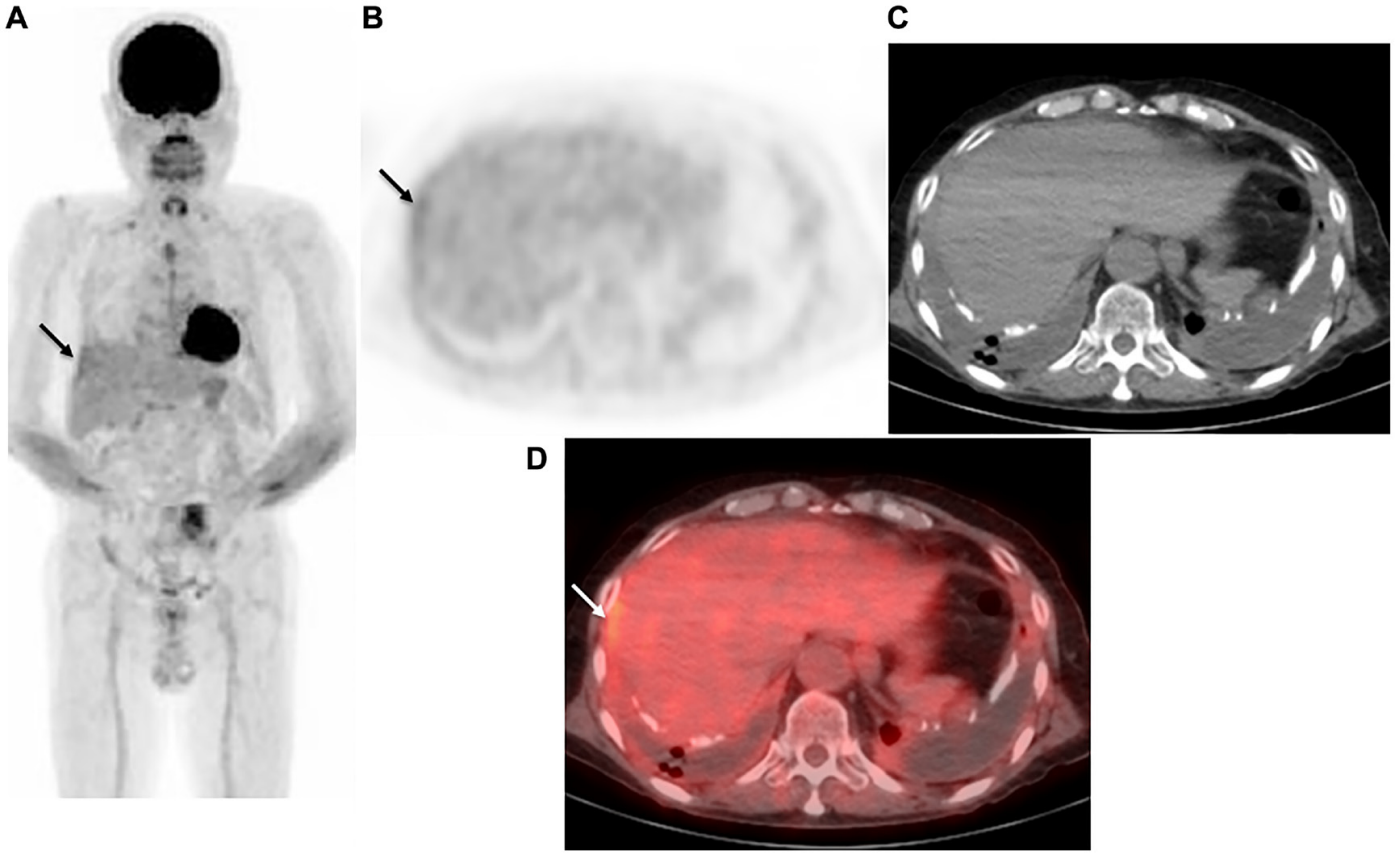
Representative case: 72-year-old male with right malignant pleural mesothelioma (epithelial type). (A) Maximum intensity projection (MIP) from FDG-PET, (B) axial FDG-PET, (C) axial CT, and (D) fused FDG-PET/CT. In the bilateral pleural cavity, pleural thickening, plaque, and calcification with effusion were seen, with weak FDG uptake (SUVmax 2.56) observed in the right thickened pleura (arrow). The case was diagnosed as negative with protocol B (grade 2). In contrast, protocol C and the AI models (protocol A with output value of 0.407, protocol D with output value of 0.649) indicated a positive diagnosis. VATS showed the sample to be positive, a malignant pleural mesothelioma (epithelial type).
